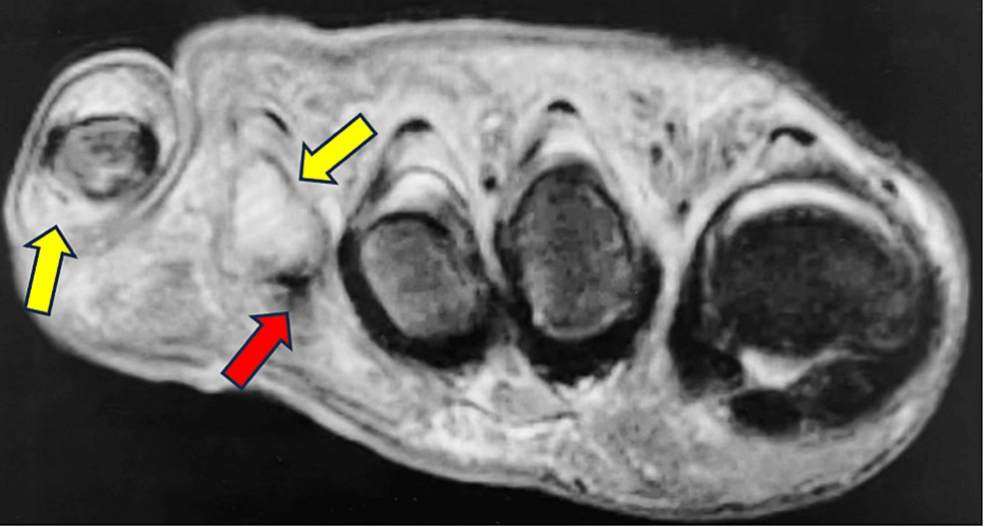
Axial MRI Without Contrast of the Right Foot An abnormal marrow signal is seen in the mid to distal fourth metatarsal/proximal phalanx, fifth metatarsal head/proximal phalanx compatible with osteomyelitis (yellow arrow). A periosteal abscess is also present on the fourth metatarsal head (red arrow).
